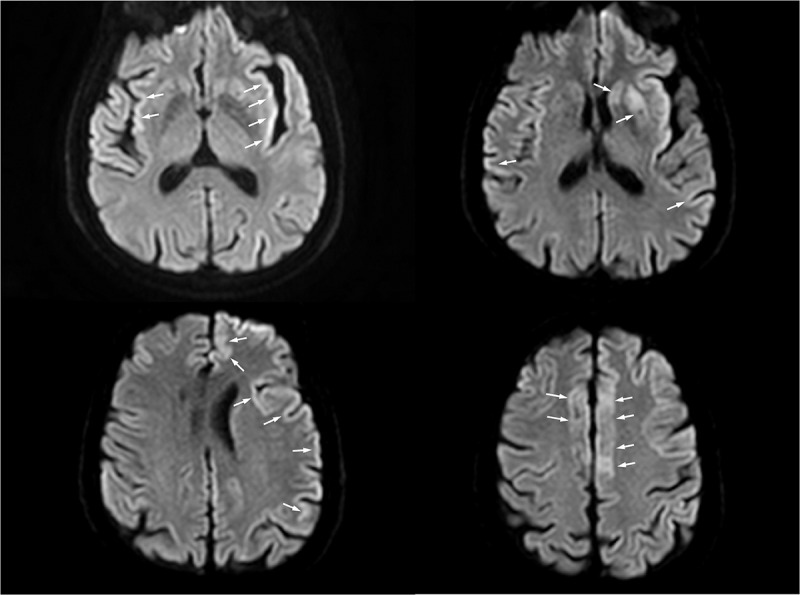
The MRI axial DWI sequence shows signal hyperintensity involving the right frontal, the left frontal and parietal cortical areas (known as cortical ribbon sign); insular cortex (especially on the left) and left corpus striatum are also involved. Areas of interest are highlighted by arrows.
